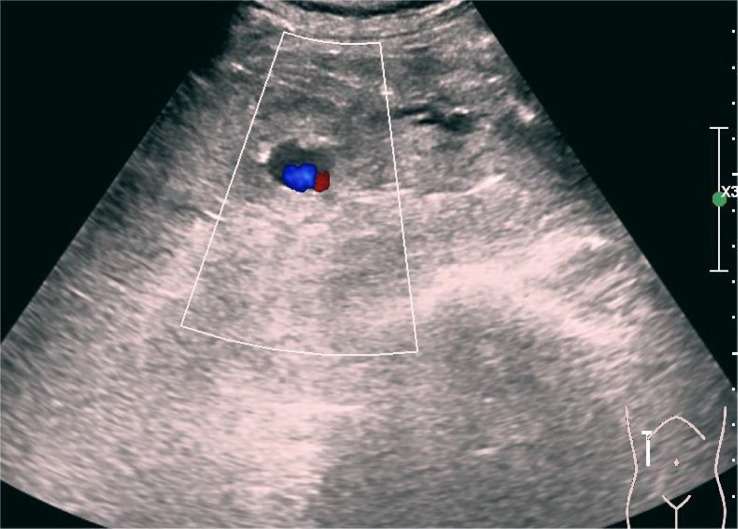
Post-puncture color Doppler ultrasound revealing hemorrhagic foci (transverse section).
